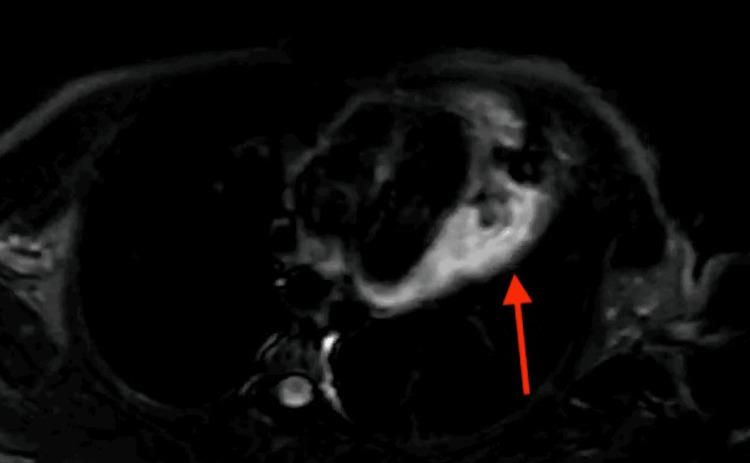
Cardiac MRI demonstrating a pericardial mass (red arrow) with hyperintense signal on late gadolinium enhancement.
